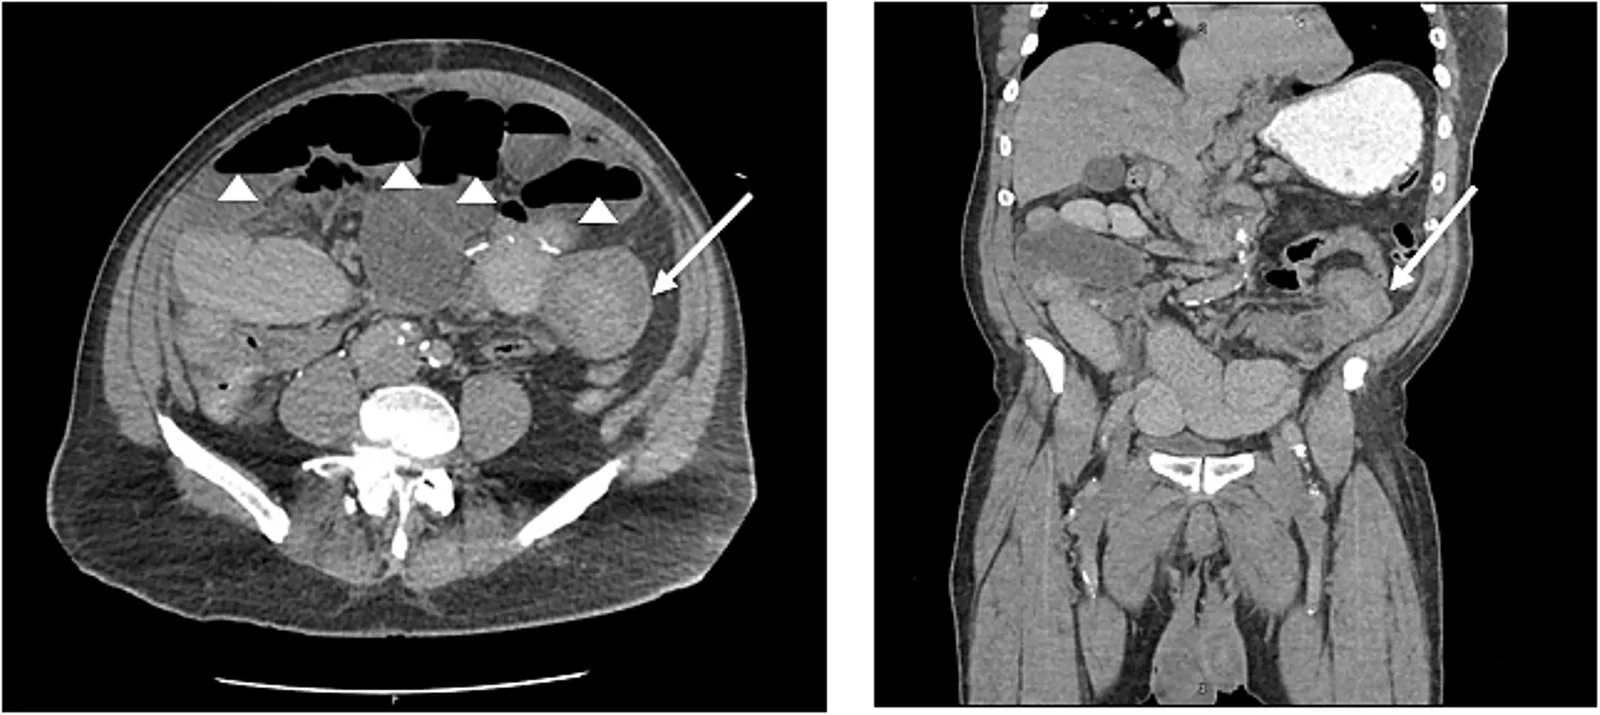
CT AP with oral and intravenous contrast: Axial view showed small bowel dilation with multiple air-fluid levels (arrowheads) indicating a small bowel obstruction. Axial and coronal view revealed and intussusception in the left lower quadrant (arrows).
